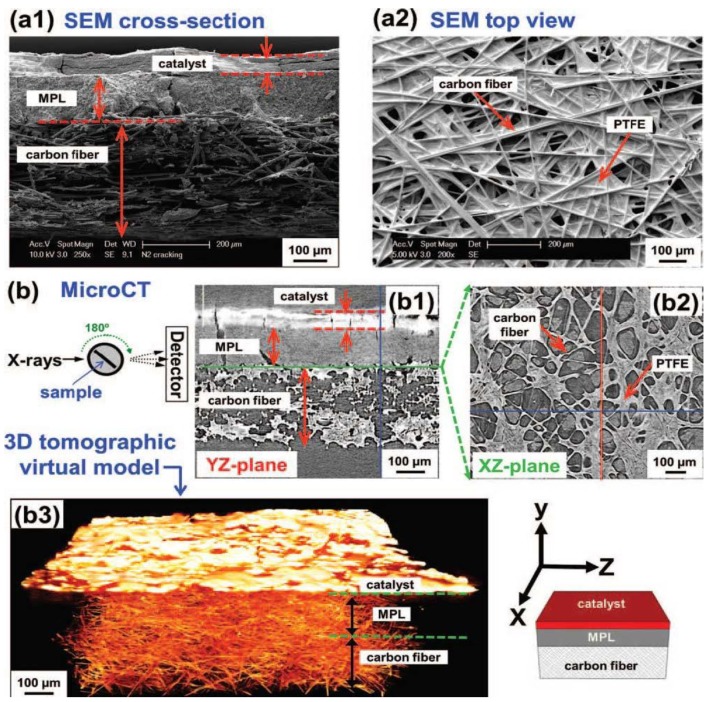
Comparison of the information obtained by traditional SEM cross-section analysis and micro-computed tomography. Reprinted with permission from [34]. Copyright 2012, The Electrochemical Society.
